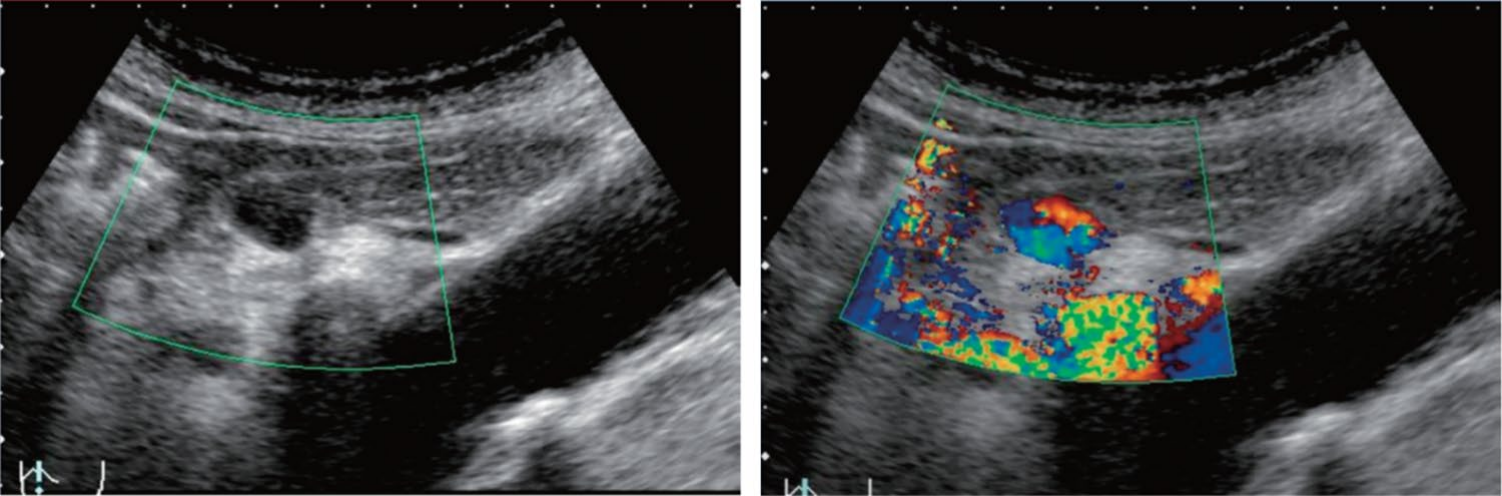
Other findings. Vascular abnormality (A cystic lesion is observed in the pancreas body by the B-mode, and an internal blood flow signal is present on color Doppler.). Left: B-mode; right: color Doppler. Category 2, Assessment D2
